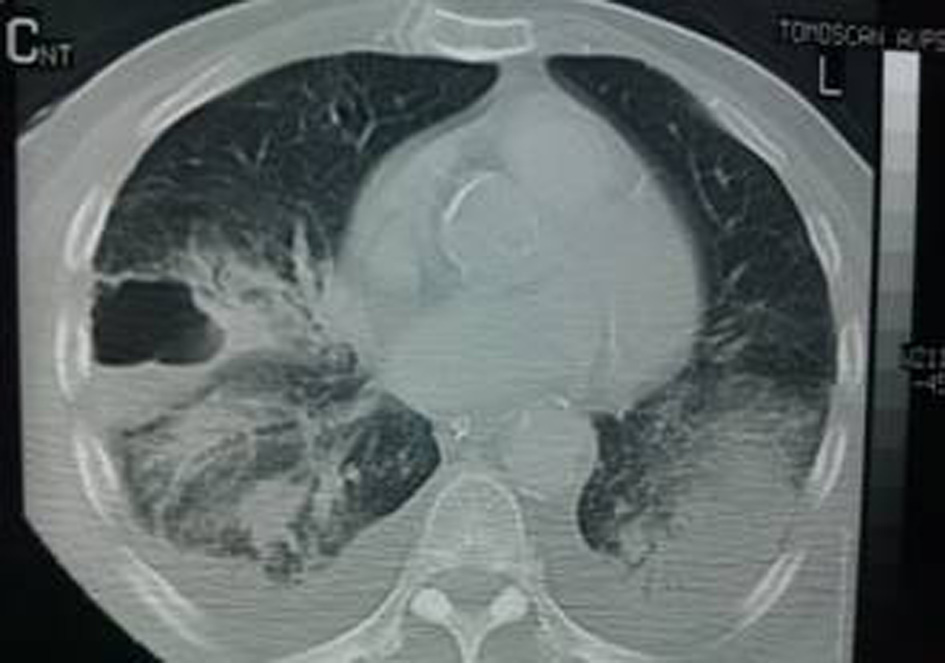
CT imaging of the lung abscess.
